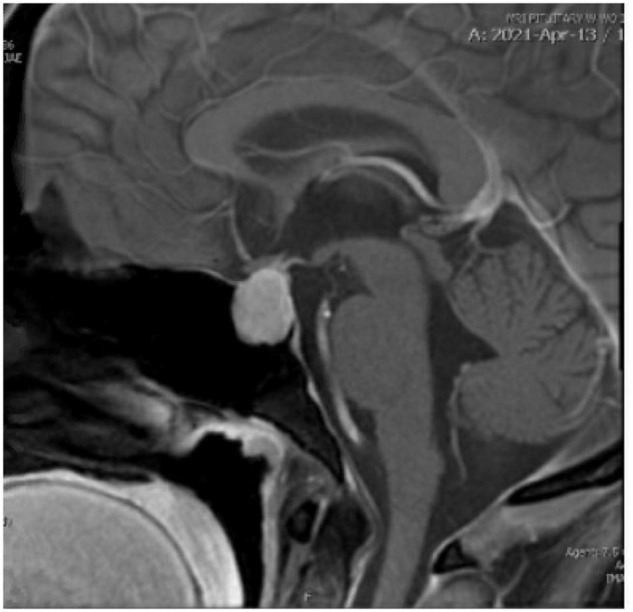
The patient’s initial MRI with pituitary protocol.
